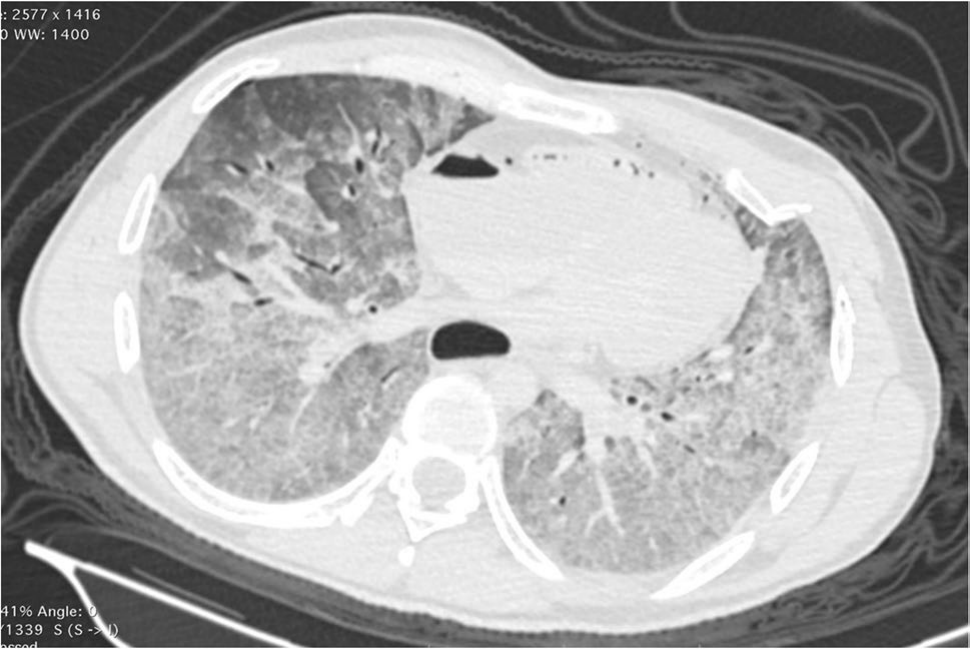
CT image showing massive edema of the lungs
